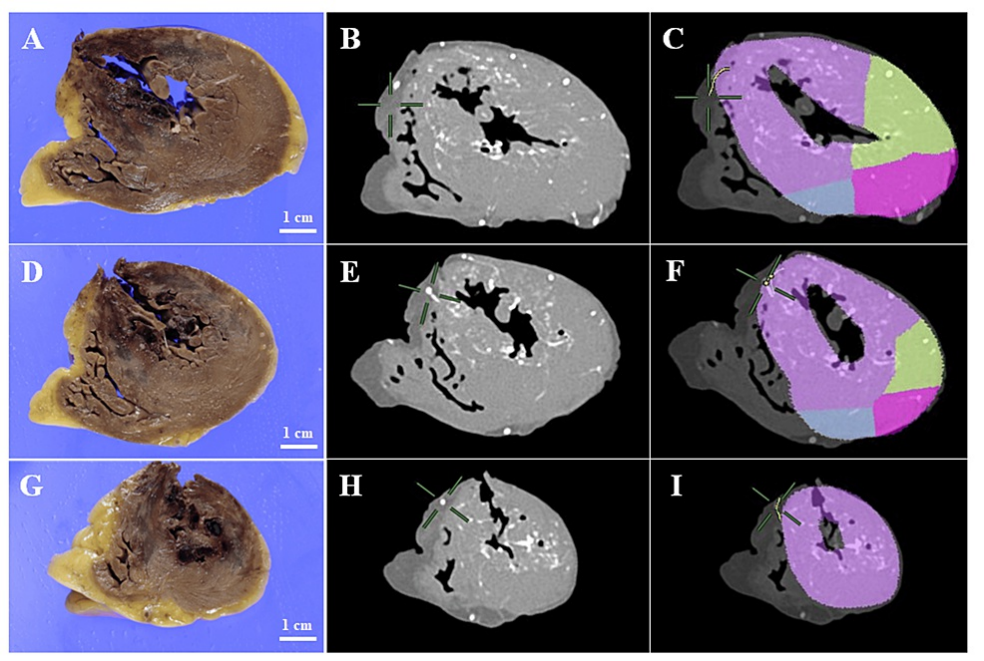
Comparison of macroscopic observation with postmortem computed tomography angiography (PMCTA) images. (A) Cross-section corresponding to the blue dashed line in Figure 2B. (B) Corresponding PMCTA image to panel A. (C) Computed tomography (CT) cross-section corresponding to panel B, with color coding of the perfusion territories: purple represents the ischemic region predicted based on the LAD stenosis, green indicates the perfusion territory of the LAD, pink indicates the perfusion territory of the LCX, and blue indicates the perfusion territory of the RCA. (D) Cross-section corresponding to the yellow dashed line in Figure 2B. (E) Corresponding PMCTA image to panel D. (F) CT cross-section corresponding to panel E, with color coding of the perfusion territories. (G) Cross-section corresponding to the green dashed line in Figure 2B. (H) Corresponding PMCTA image to panel G. (I) CT cross-section corresponding to panel H, with color coding of the perfusion territories. LAD: Left anterior descending artery
